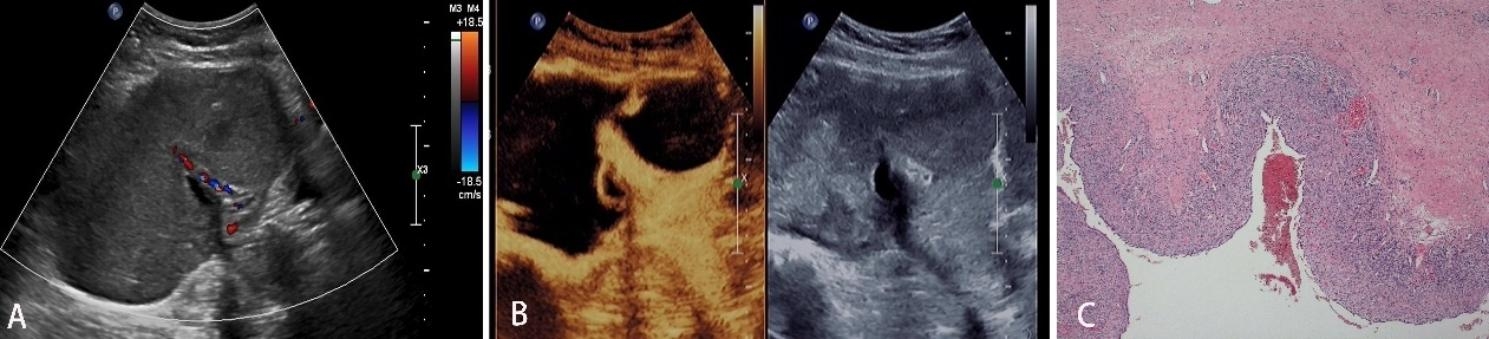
Transabdominal ultrasound and pathological image of a 23-year-old patient with a right adnexal mass. A: Conventional ultrasound indicated that the maximum diameter of the mass was 14.2 cm, irregular in shape and solid in appearance; CDFI showed the strip-shaped blood flow signal in the center of the mass, which was classified as 5 by O-RADS. B: CEUS 20s showed high annular enhancement at the rim of the mass, and there was no enhancement inside except for the separation, which was downgraded to 3 using O-RADS combined with CEUS. C: Pathological results indicated a right ovarian endometriosis cyst (HE × 40)
